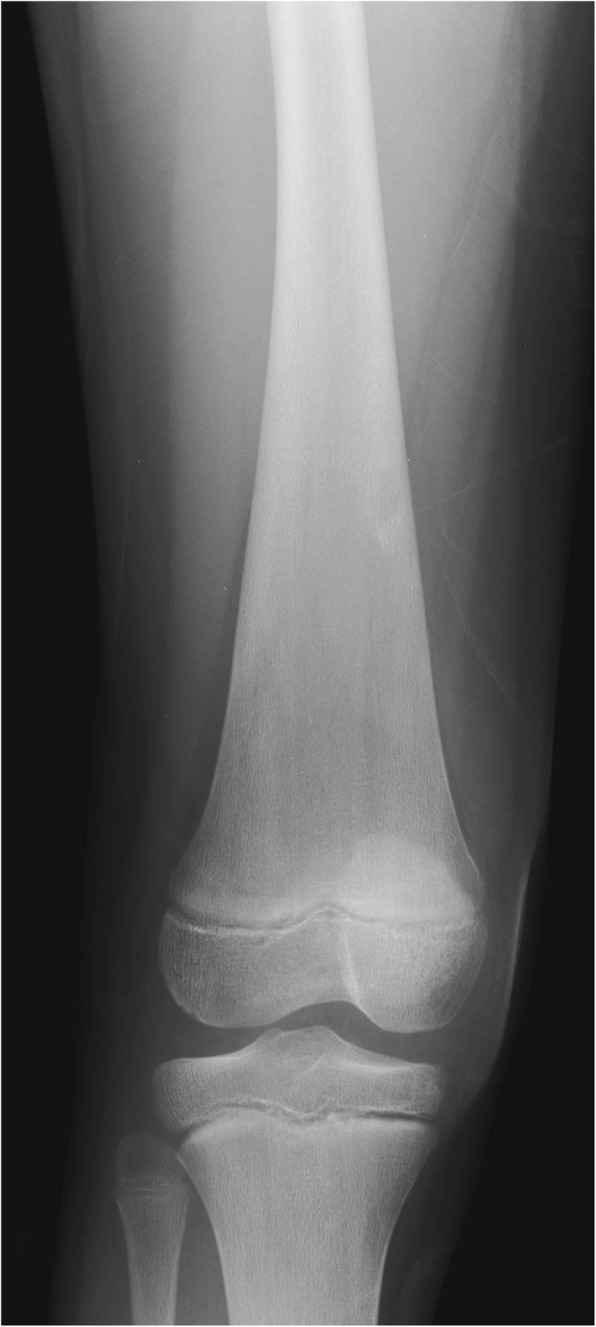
Erlenmeyer flask deformity. Frontal radiograph of the right knee in a 12-year-old-male Gaucher disease patient with widening of the distal femoral diaphysis and metaphysis resulting in Erlenmeyer flask deformity
